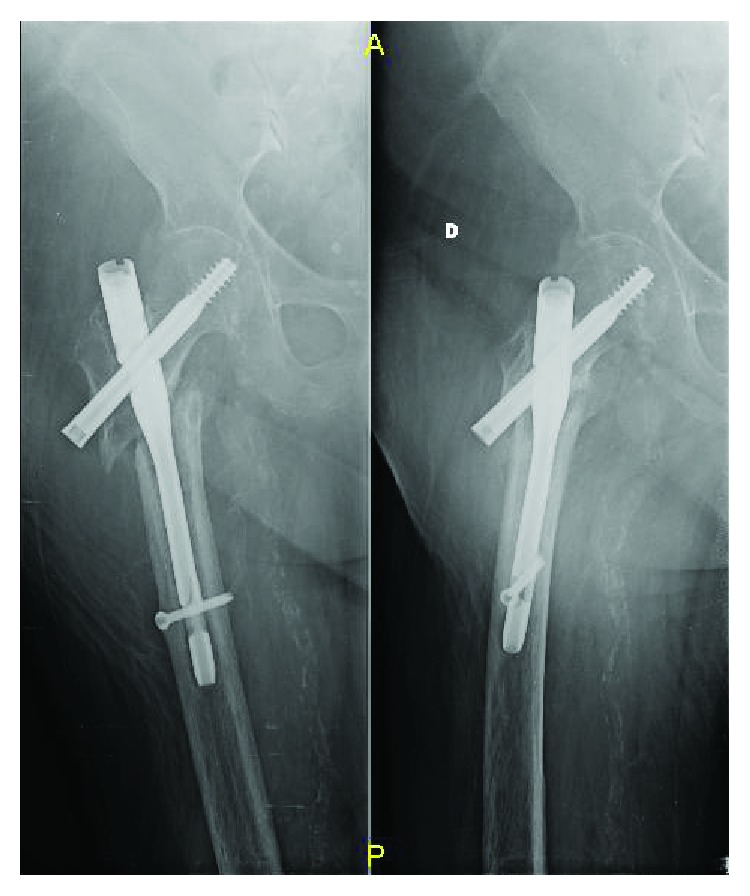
X-rays done in other hospital after 2 years of follow-up showed that the second incomplete breakage was through the barrel for the lag screw while the first incomplete breakage was on the hole for the distal static screw. The orthopaedic surgeon did still not talk about nail dynamization or nail remotion and revision to the patient.
